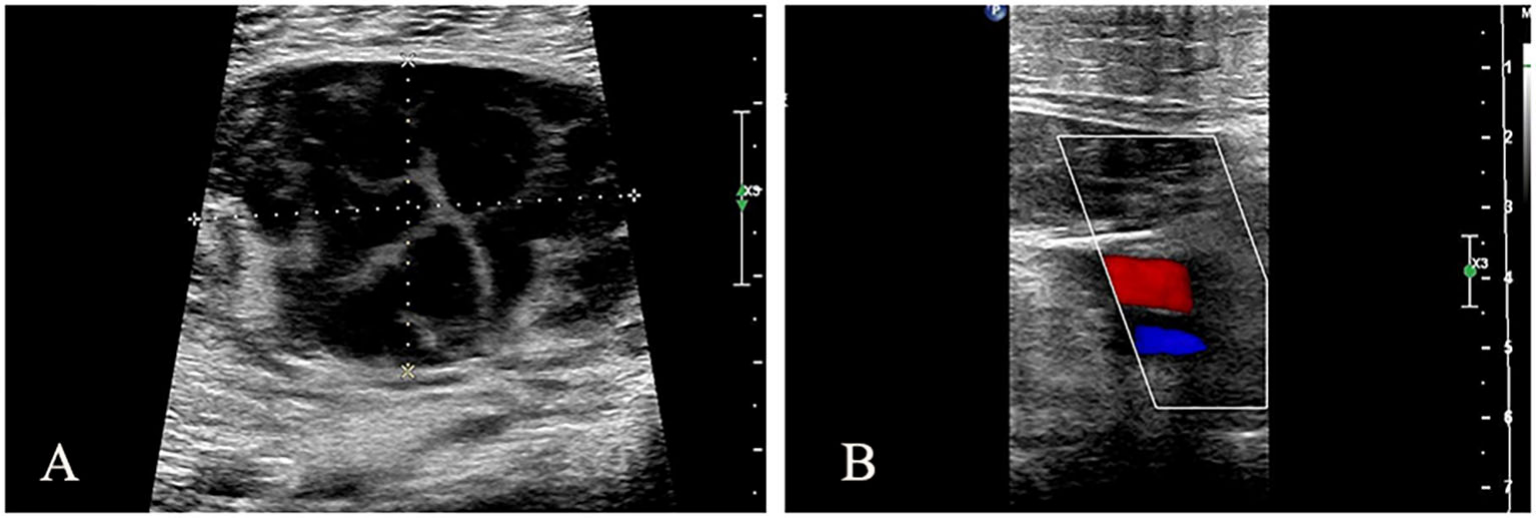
Ultrasound Doppler image of left lower extremity. (A) Hypoechoic area in left thigh muscle layer, approximately 51 × 36 mm, with clear boundary and uneven internal echo. (B) CDFI: No obvious blood flow signal was observed.
